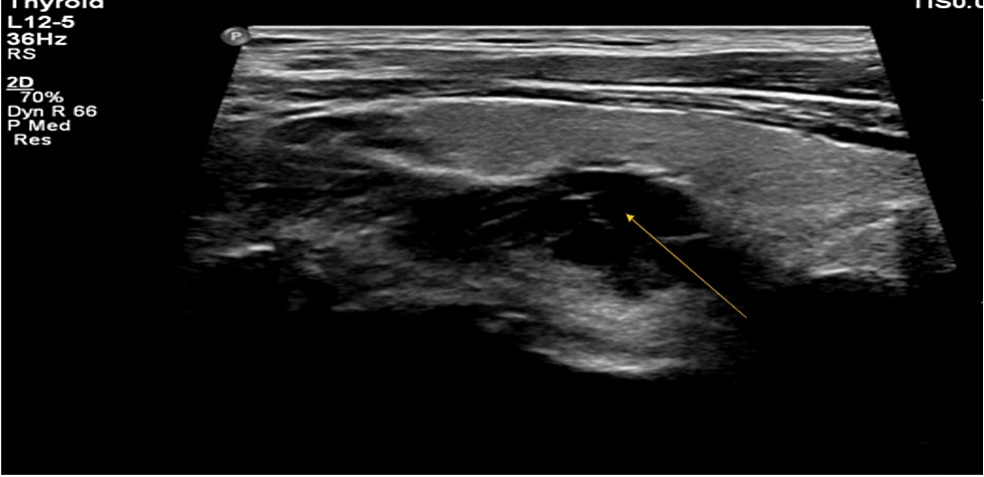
Thyroid Lobe (R) Ultrasound Neck sonogram highlighting anechoic lesion with septa in the right thyroid lobe
